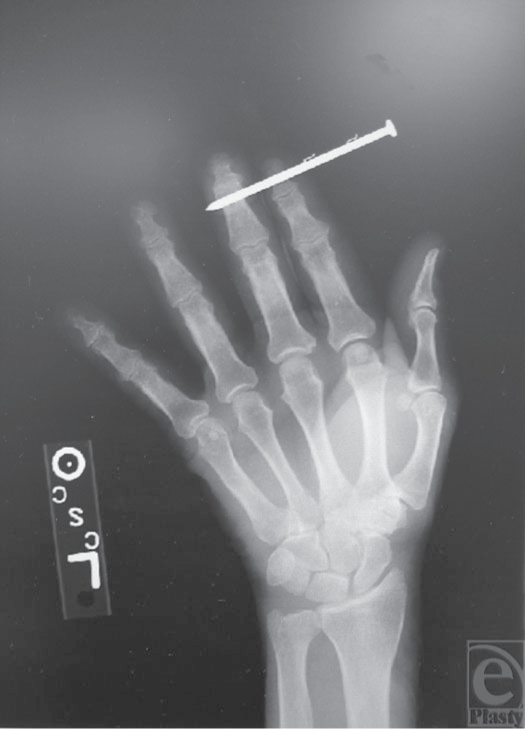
X-ray film demonstrates no bony injury with barbs located outside the soft tissue of the fingers.
